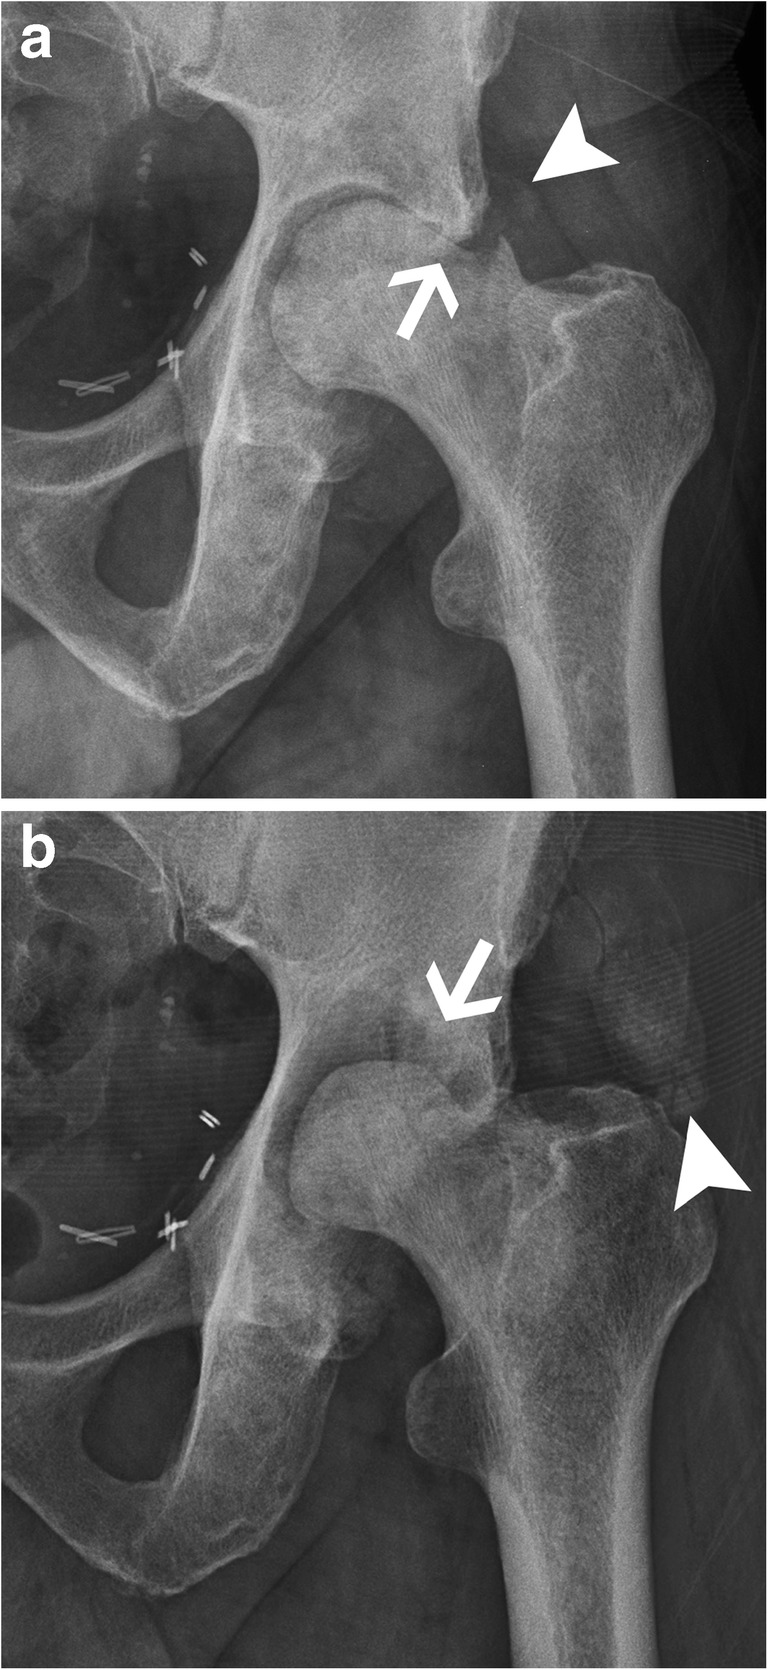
Rapid degenerative arthropathy. (a) Anteroposterior radiographs initially and (b) 3 months later show progressive bone lysis (arrows) and periarticular calcifications (arrowheads)
